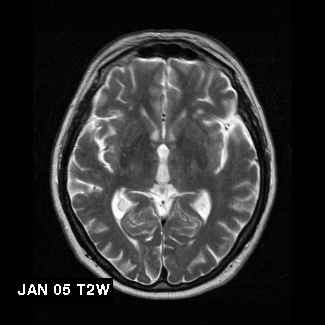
Initial radiological findings in Case 7: T2 weighted MRI scans of Case 7 showing extent of white matter disease in January 2005
